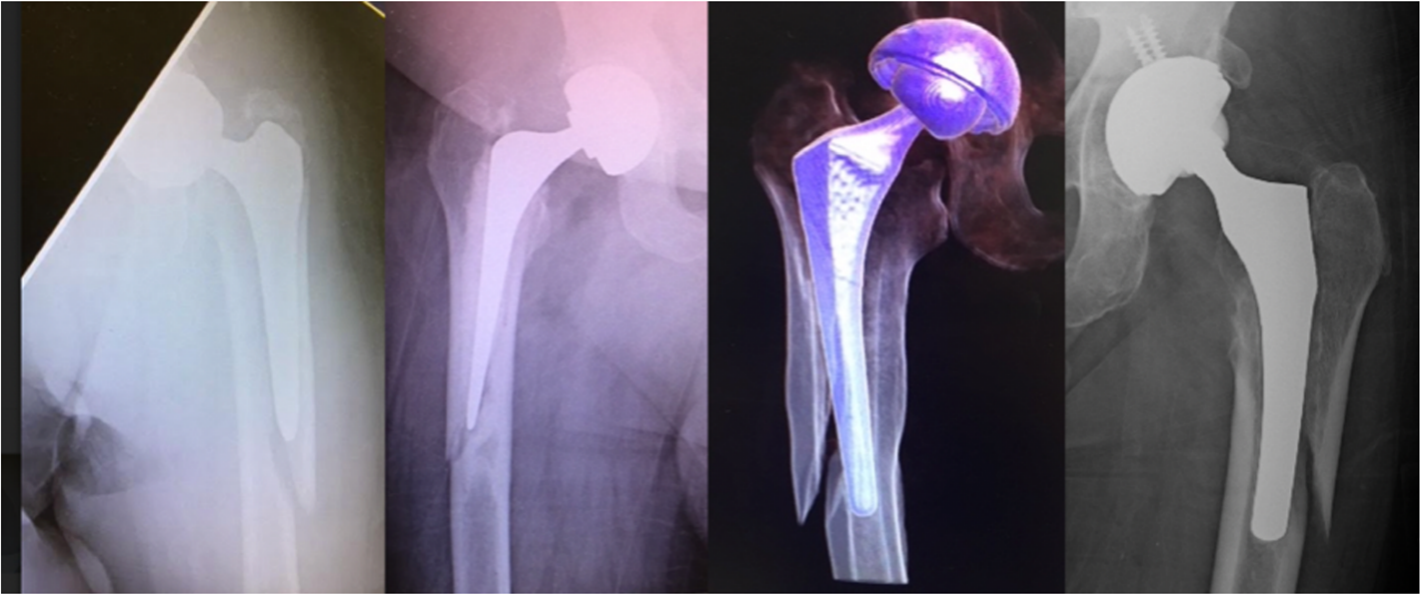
Reverse clamshell fracture pattern. In these radiographs, further examples of the reverse clamshell fracture pattern are shown in both cemented and uncemented stems displaying the typical fracture pattern involving the lateral cortex only with preservation of the medial cortex
